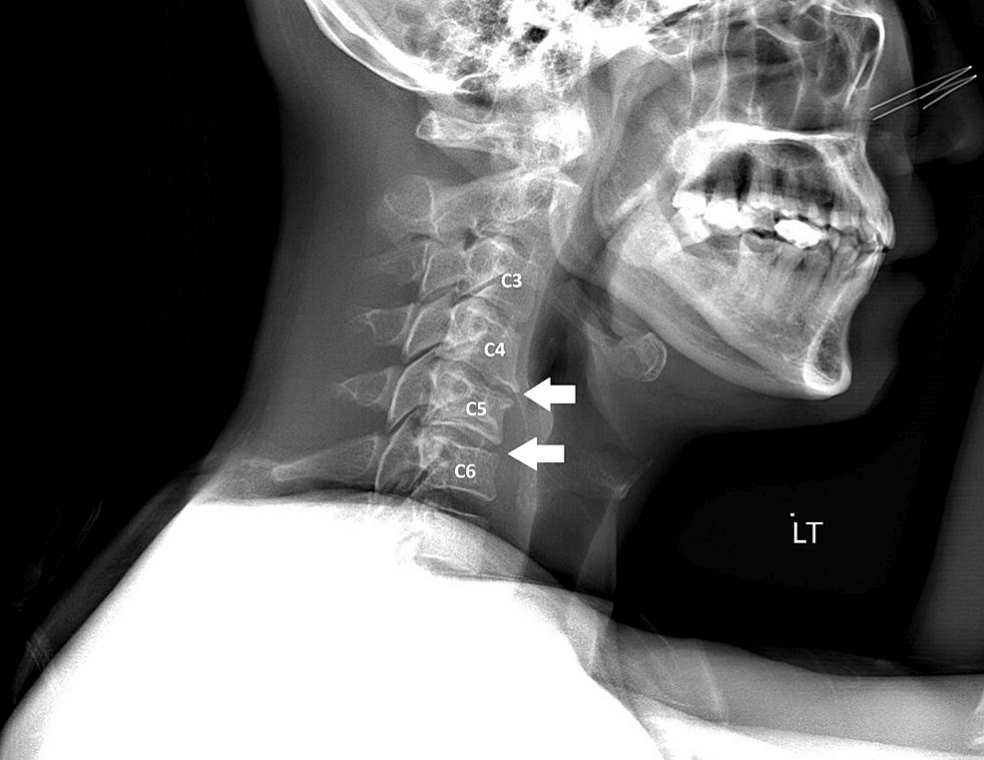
Cervical radiograph. Cervical radiograph demonstrating multilevel degenerative changes. There are anterior marginal osteophytes noted along the cervical spine. Intervertebral disc space narrowing is present at levels C2–C6, indicative of disc degeneration. Of note, the greatest degree of disc space narrowing is seen at C4/5 and C5/6, corresponding to the level of nerve root impingement seen on magnetic resonance imaging of the patient’s cervical. These degenerative changes involving the C5/6 segment align with the patient’s clinical presentation of right C6 radiculopathy.
